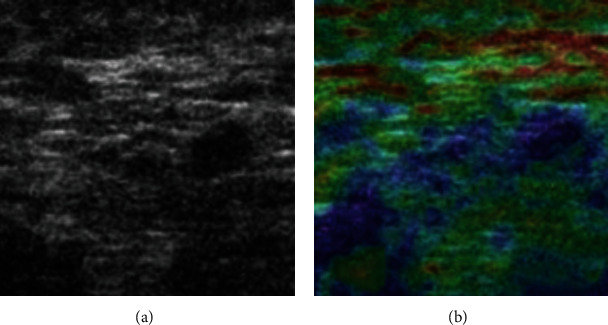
Comparison of lymph node metastases of breast cancer in different ultrasound methods. (a) Routine US; (b) UE.
